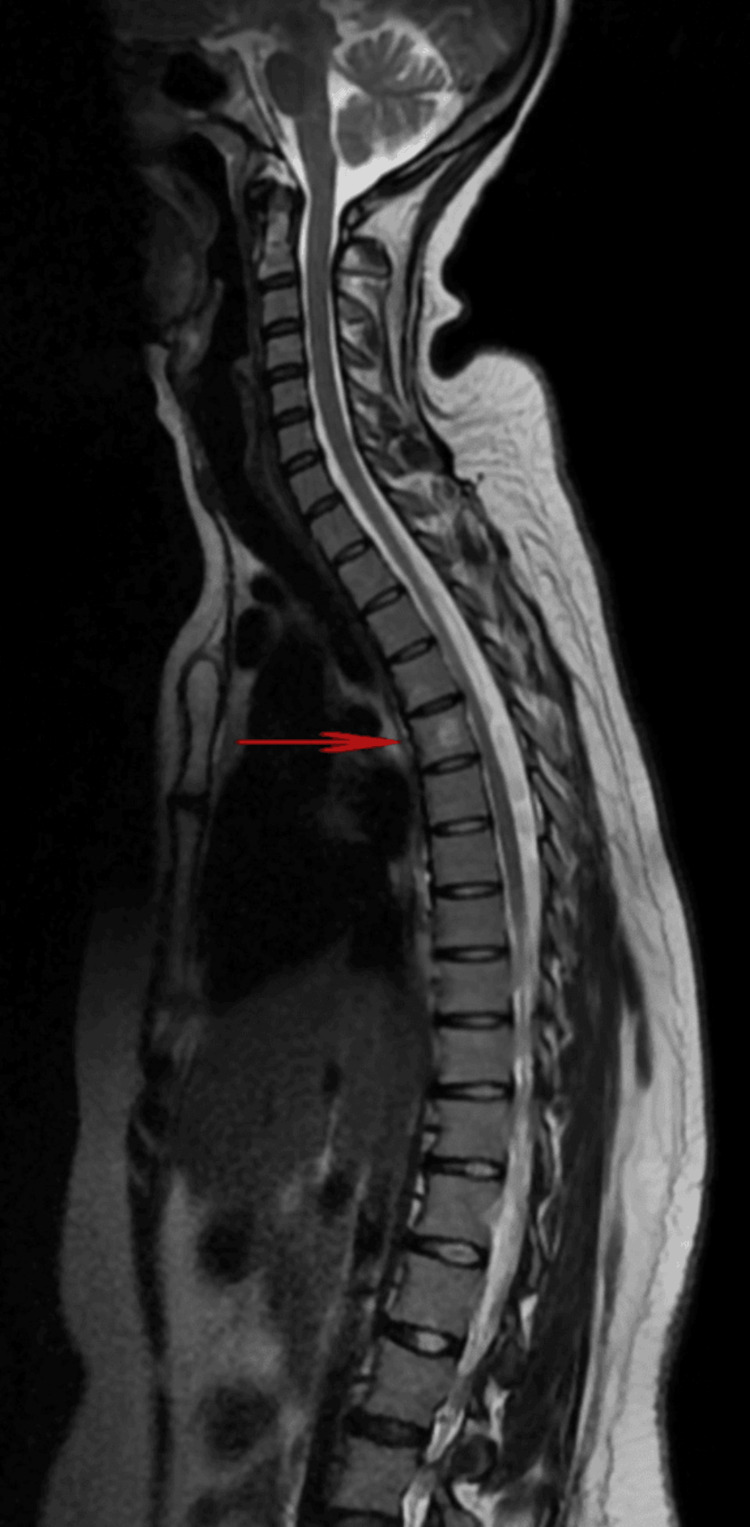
Sagittal T2 MRI of the spinal cord with mild degenerative changes
